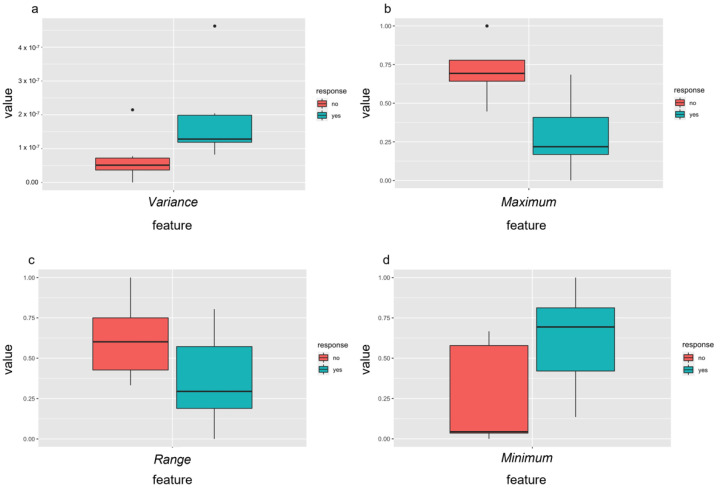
Independent radiomic features of pre-treatment MRI differentiated well between responders (yes = green) and non-responders (no = red) to percutaneous sclerotherapy using the identified features after feature selection. Response was defined as an increase in pain score of at least 20 points assessed with the SF-36 (a) or improvement of the TESS score of at least 5% (b) after the first treatment and as an achievement of at least 85 points in the SF-36 pain score (c) or of 85% or more in the TESS score (d) after repetitive percutaneous sclerotherapy.
